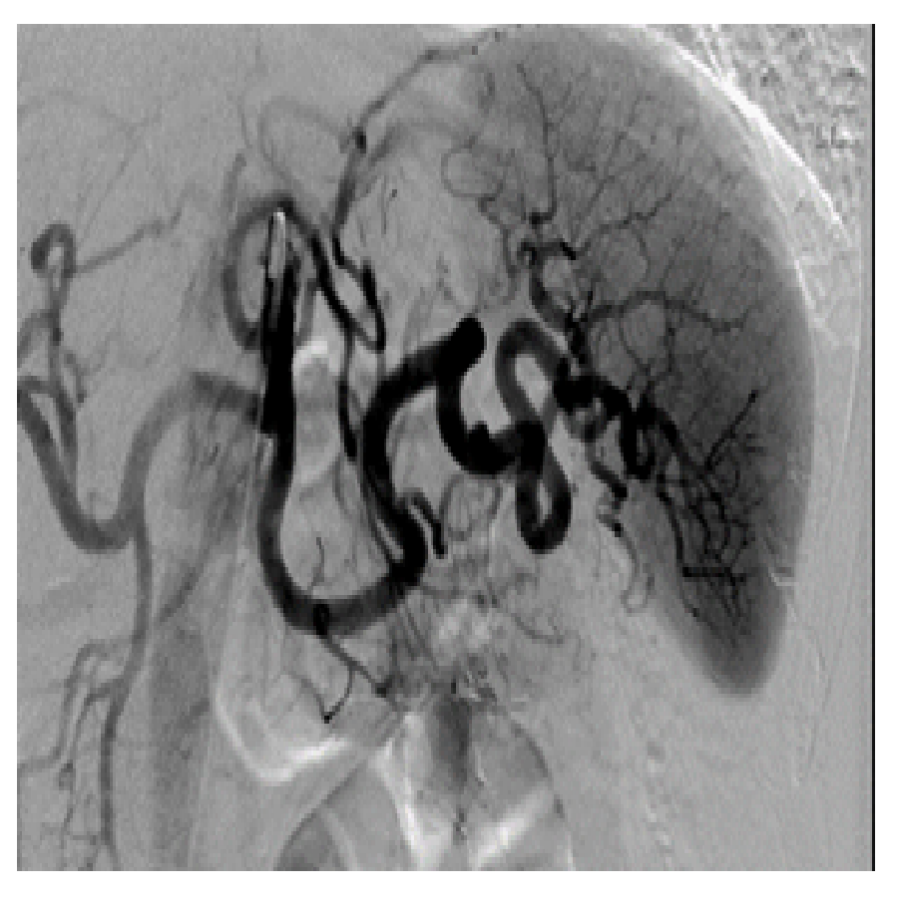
Computed tomography angiogram of celiac artery showed no evidence of active pseudocyst bleeding or vascular abnormality.
